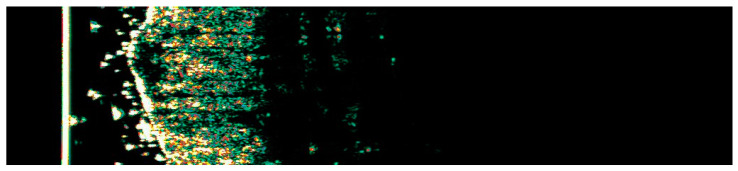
Ultrasonographic image of BCC on the temple.
